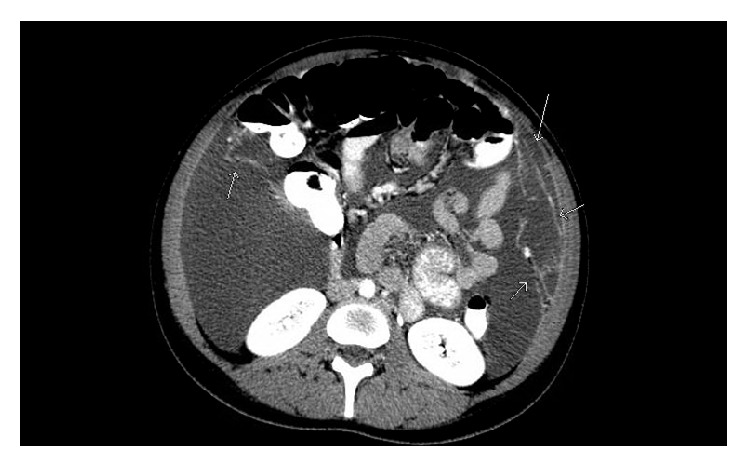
CT scan of the abdomen and pelvis revealing a significant amount of ascites and enhancing predominantly linear soft tissue densities in the right and left upper quadrant labelled by arrows.
